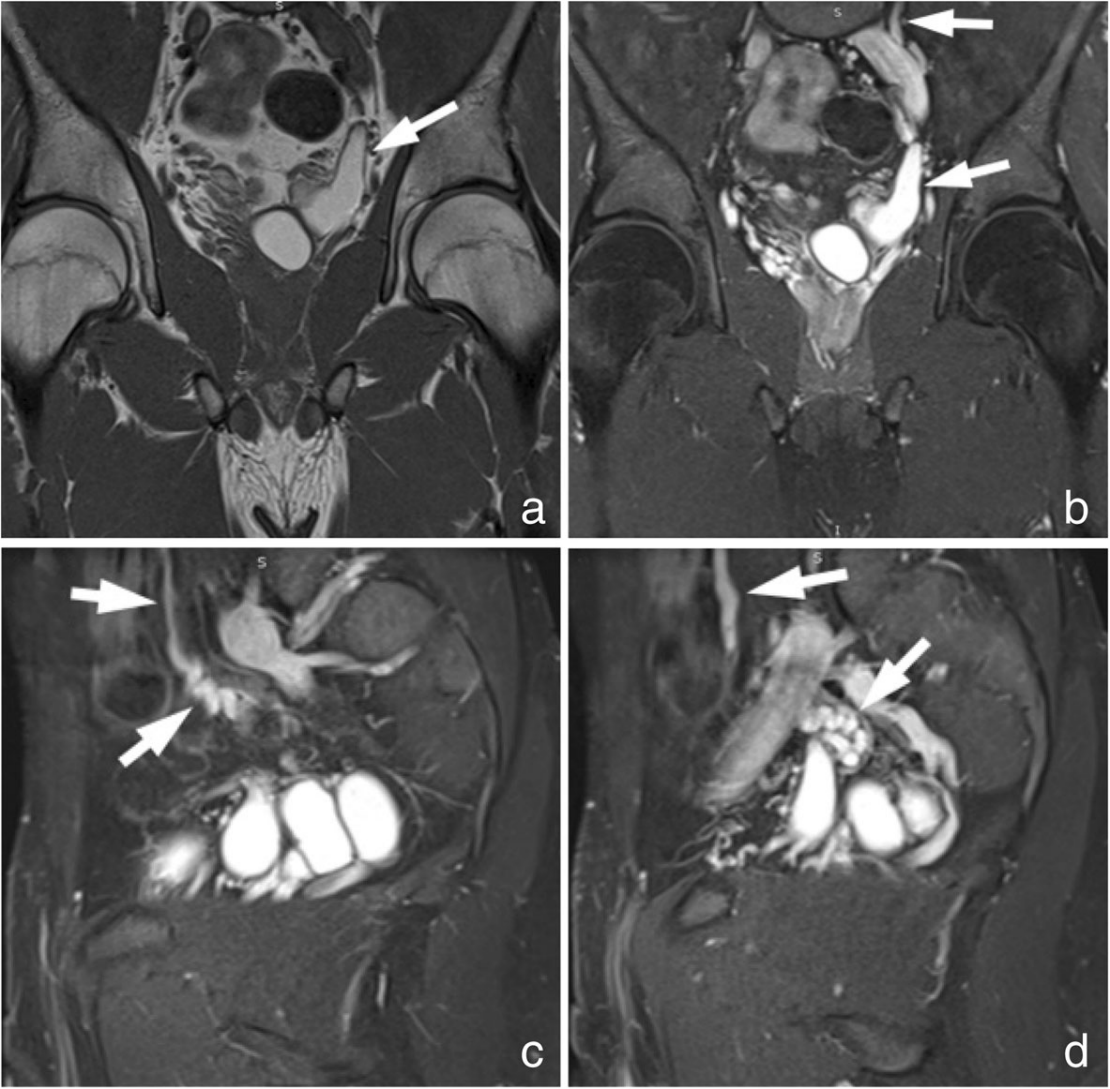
Coronal T_1_-weighted (a) and corresponding T_1_-weighted FS image after intravenous administration of contrast medium (b) depict a saccular dilated ectopic left ureter opening into the multilobulated SVC. The ectopic ureter is also observed extending centrally with a tortuous morphology on adjacent T_1_-weighted FS images after intravenous administration of contrast medium (c and d). High signal intensity in the ureter indicative of proteinaceous or hematic content is present on all MR images (arrows)
